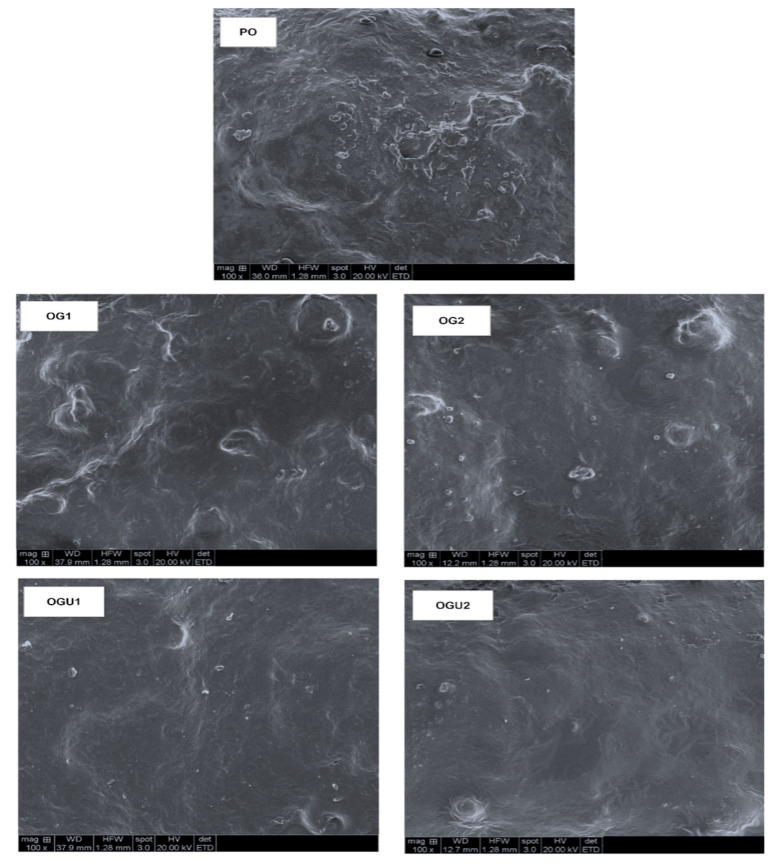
Microstructural observation of the surface of SDEW instant noodles that are fried using palm oil, palm oil–carnauba wax oleogel and palm oil–carnauba wax oleogel with ultrasonication treatments. Note: PO represents palm oil; OG1 represents palm oil–carnauba wax (5 g/100 g); OG2 represents palm oil–carnauba wax (10 g/100 g); OGU1 represents palm oil–carnauba wax (5 g/100 g) homogenized with ultrasonication; and OGU2 represents palm oil–carnauba wax (10 g/100 g) homogenized with ultrasonication.
